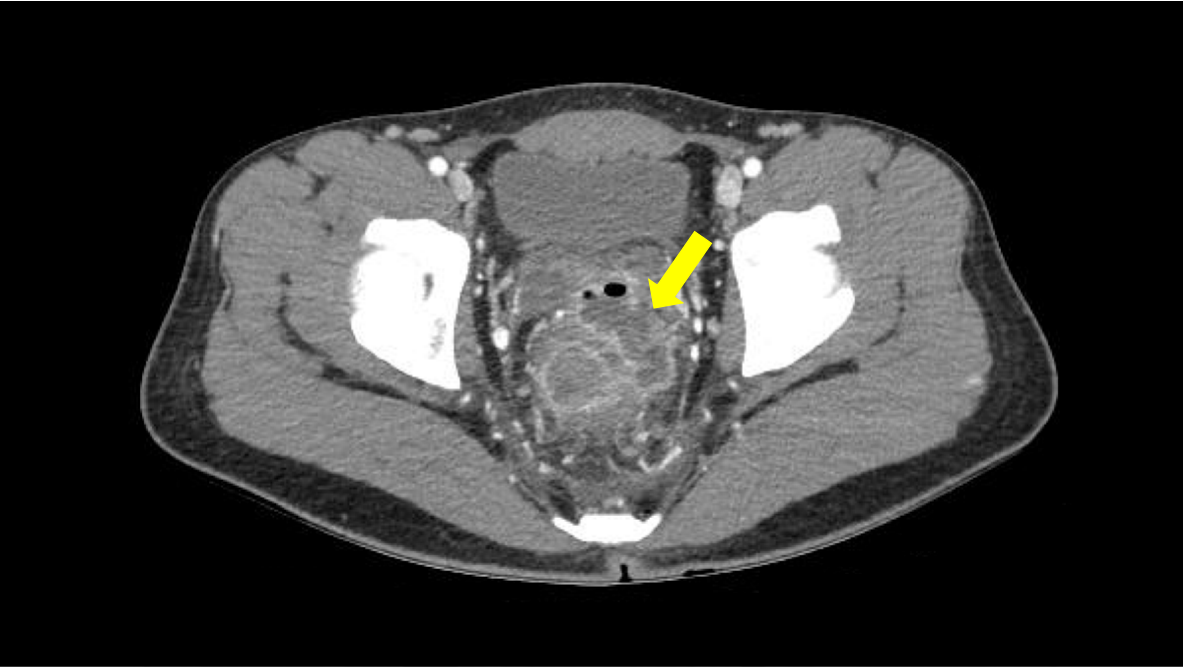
Abdominal computed tomography on hospital day 15
